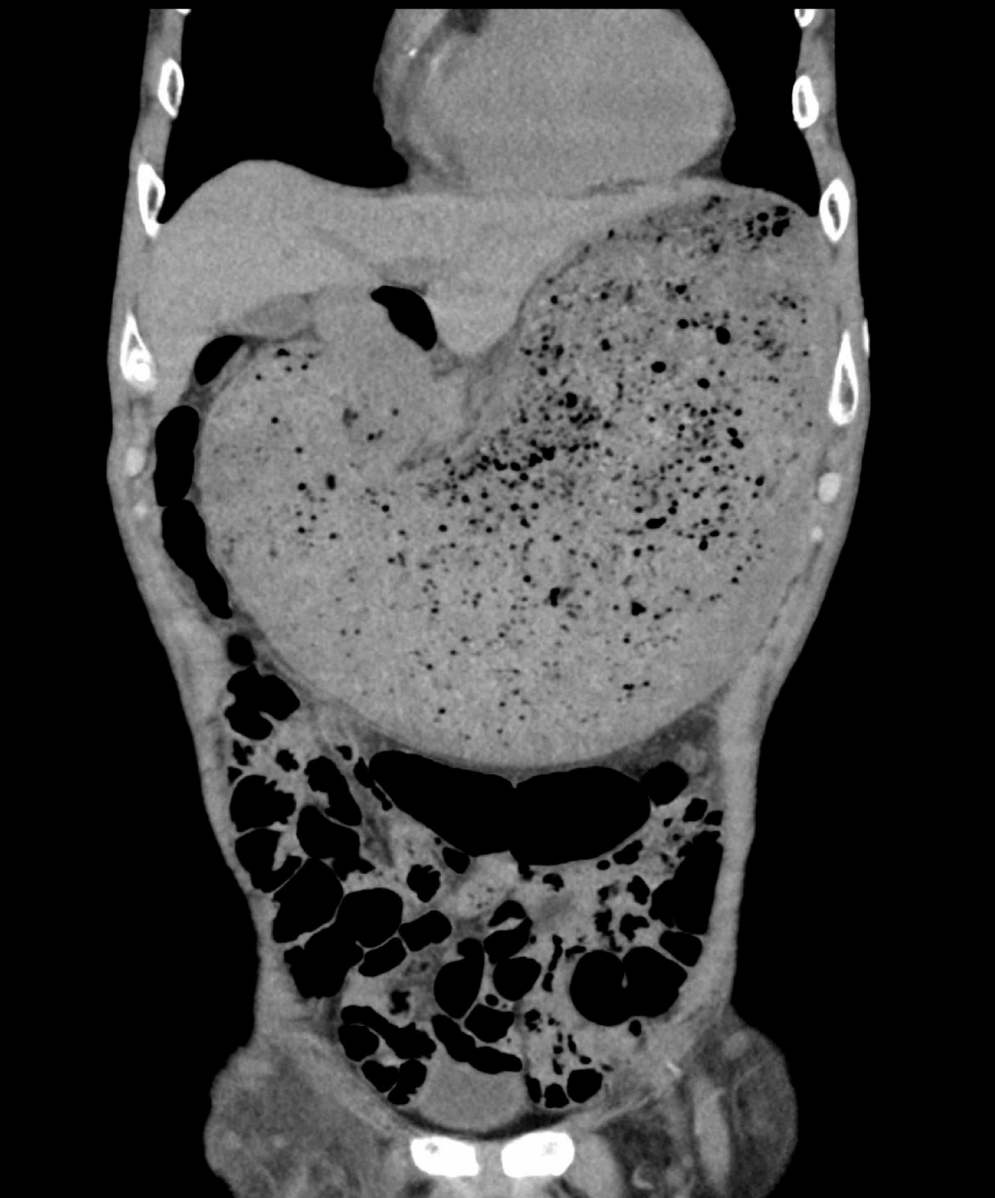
Plain abdominal computed tomography shows gastric distension and massive accumulation of food residues.
